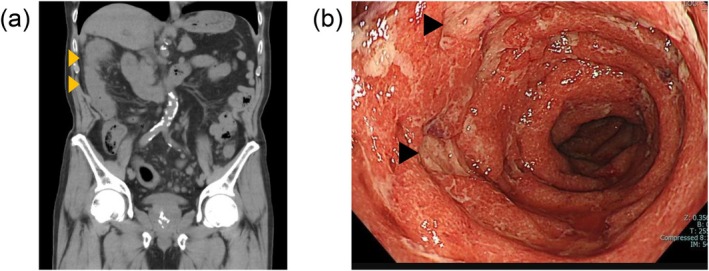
Imaging findings at admission. (a) Abdominal plain computed tomography showing bowel wall thickening throughout the colon (orange arrows). (b) Lower gastrointestinal endoscopy showing Grade 3 ulcerative lesions throughout the colon (black arrows).
